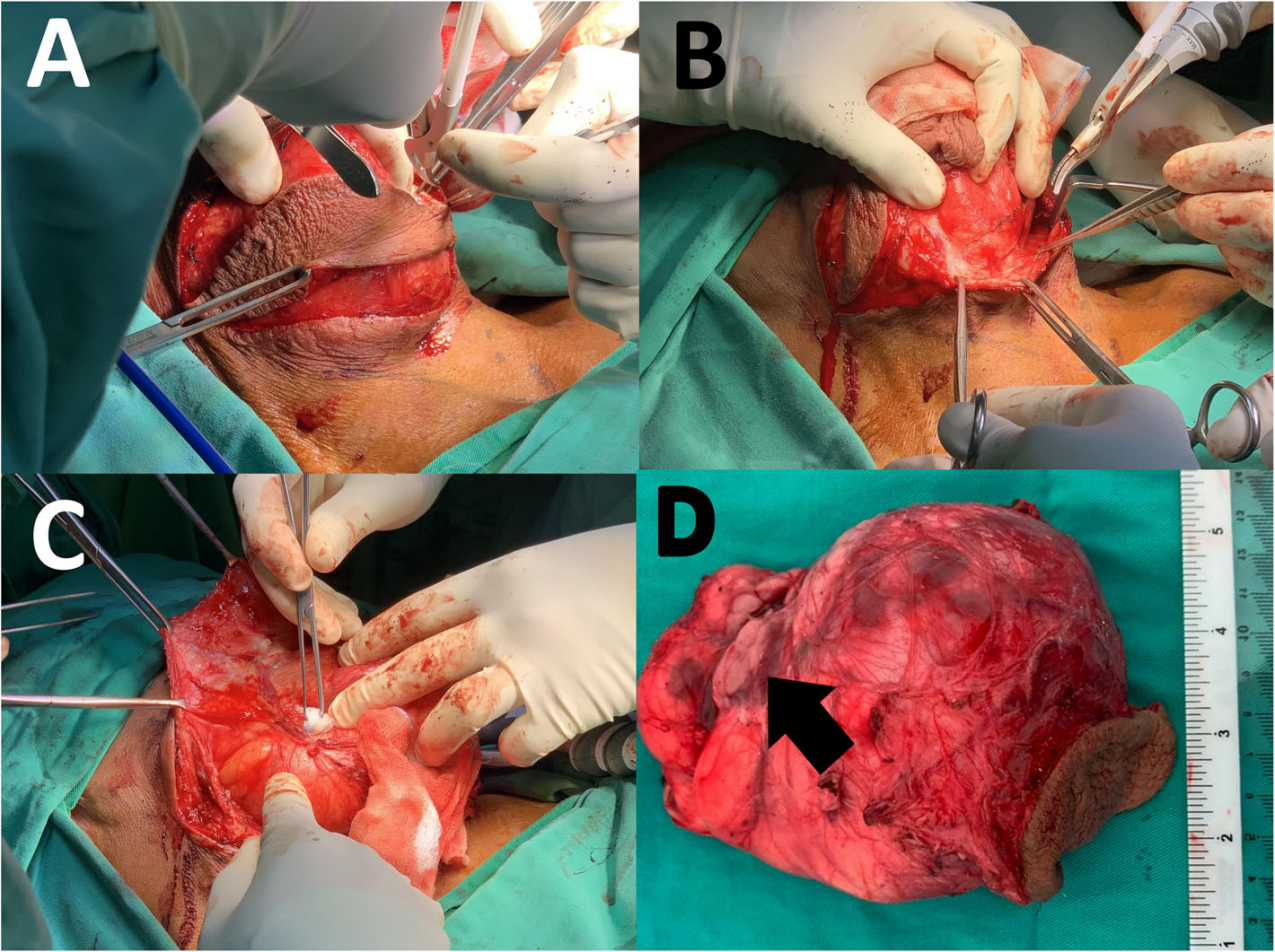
a Elliptical incision made and a portion of skin containing the previous FNAC tract was removed en-bloc with the tumour. This portion of skin is used to manipulate the tumor without handling the tumor itself besides providing better cosmesis by reducing excess skin. b Enucleation of the lesion with its capsule by creating a lower flap. An ultrasonic dissection device was used to seal and divide the peritumoral vessels and achieve haemostasis. c Creation of an upper flap and dissection of the engorged peritumoral vessels is demonstrated here. d Macroscopic appearance of the lesion showing a lipomatous tumour with intact capsule measuring 10 × 12 cm in size. The right submandibular gland was excised en bloc (black arrow)
